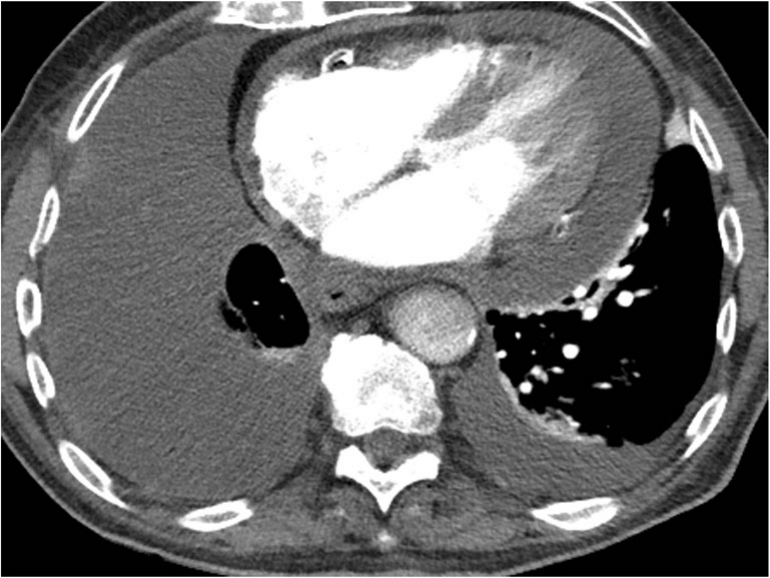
Pericardial tamponade on CECT. Axial CTA shows moderate pericardial effusion and heterogeneous enhancement of descending aorta with blood contrast layering. This is indicative of poor stroke volume from tamponade
